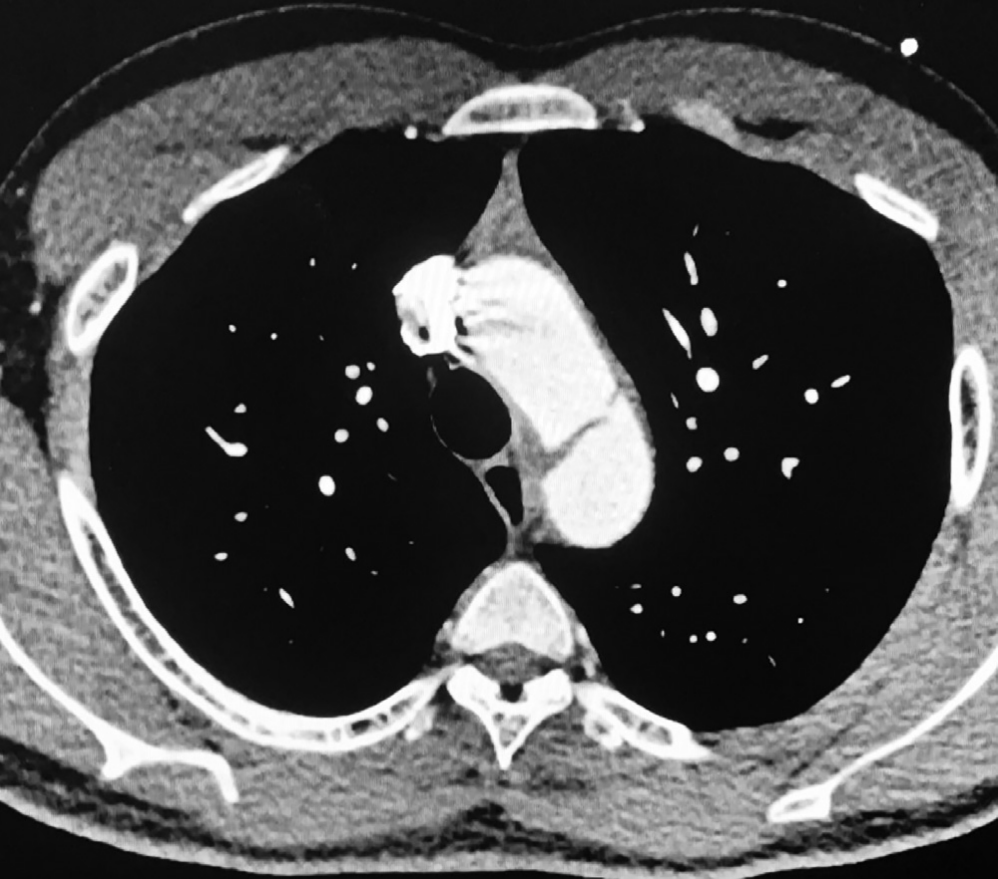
Aortic Dissection seen on CT Scan.
